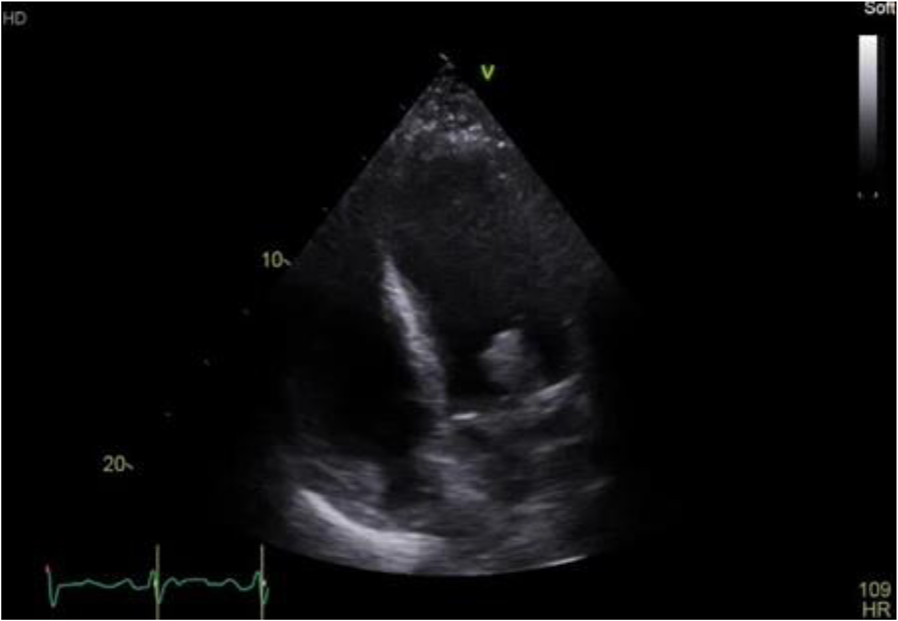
Transthoracic Echocardiogram revealing large, mobile vegetation on mitral valve
